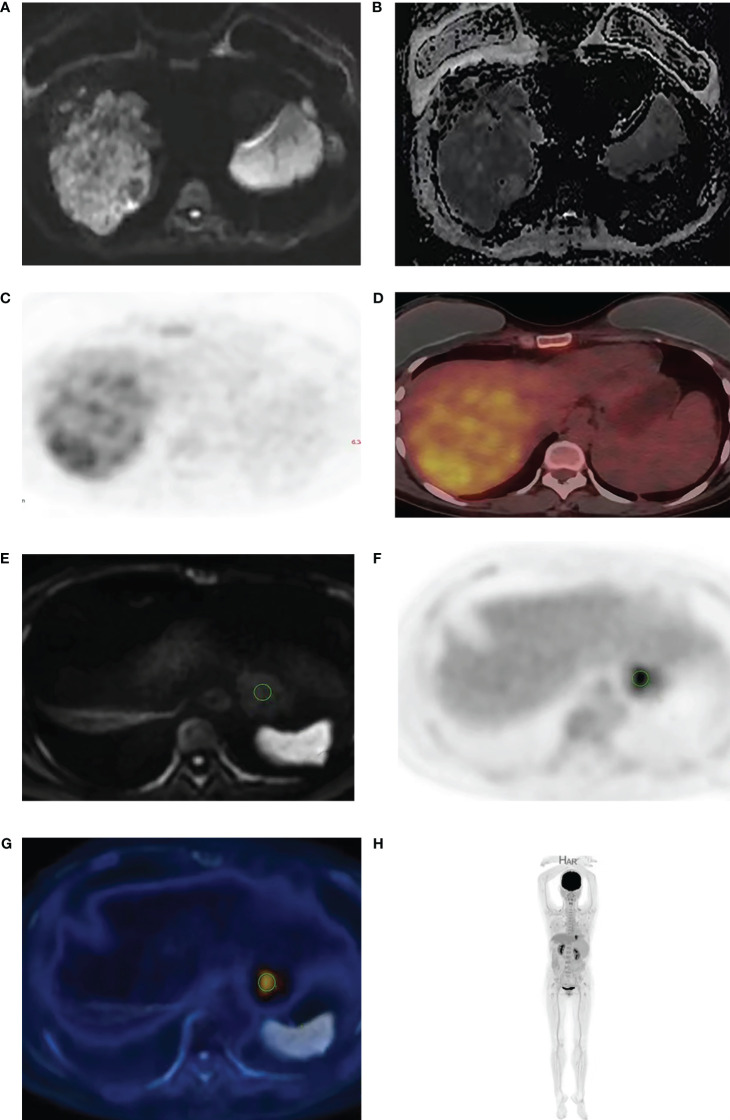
A 53-year-old man with HCC. The high b value (b=2000) diffusion weighted image (A) shows high signal intensity in HCC with corresponding low signal in the ADC maps (B). ^18^F-FDG PET (C) and fused PET/DWI (D) images show strong 18F-FDG uptake. The delineation of ROI is achieved through the integration of different machines (E–H).
